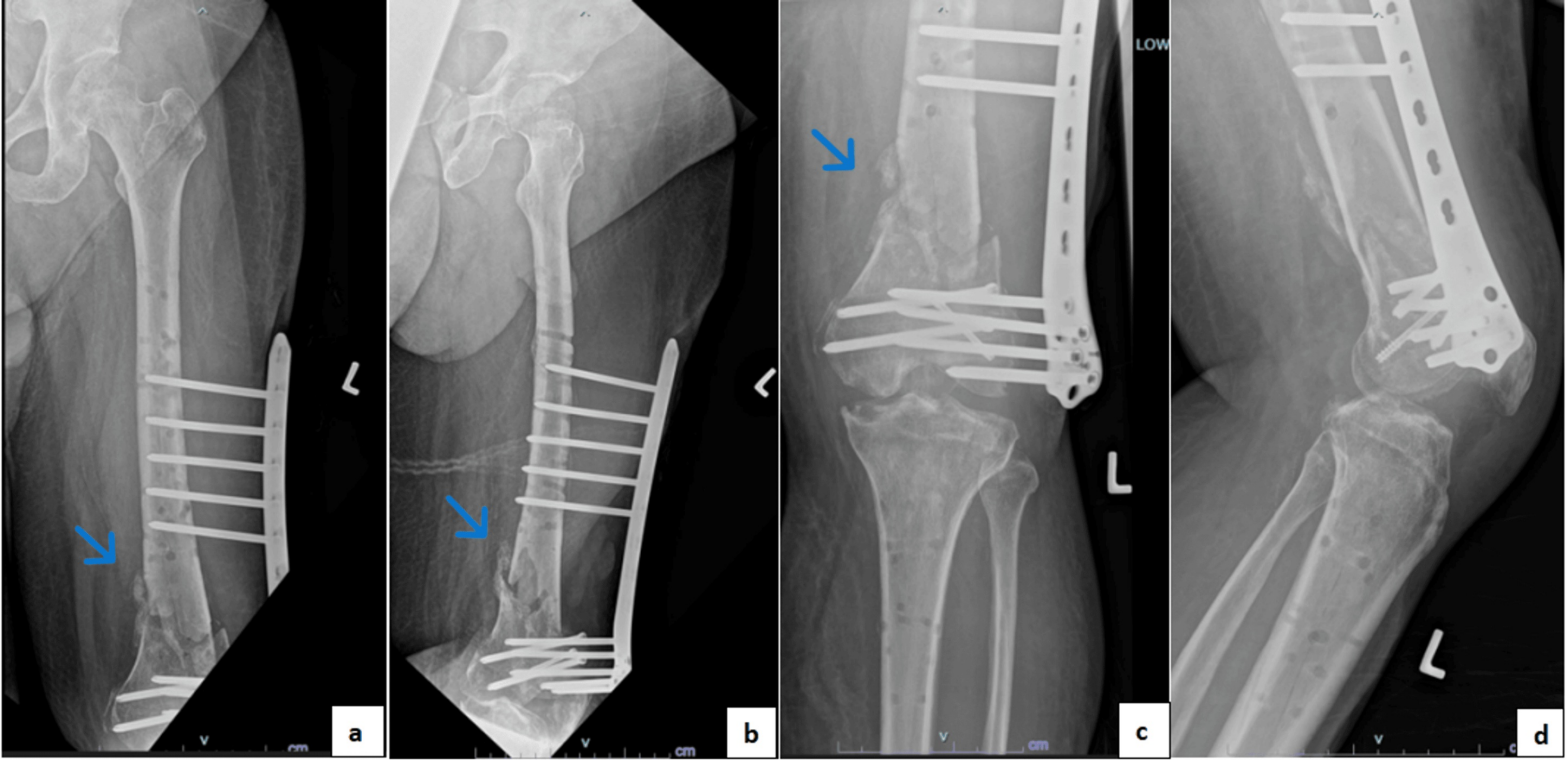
Radiographs at three months post-supracutaneous plating showing callus formation at the fracture site. (a) Femur anteroposterior view. (b) Femur lateral view. (c) Knee anteroposterior view. (d) Knee lateral view. The blue arrows show callus formation.
